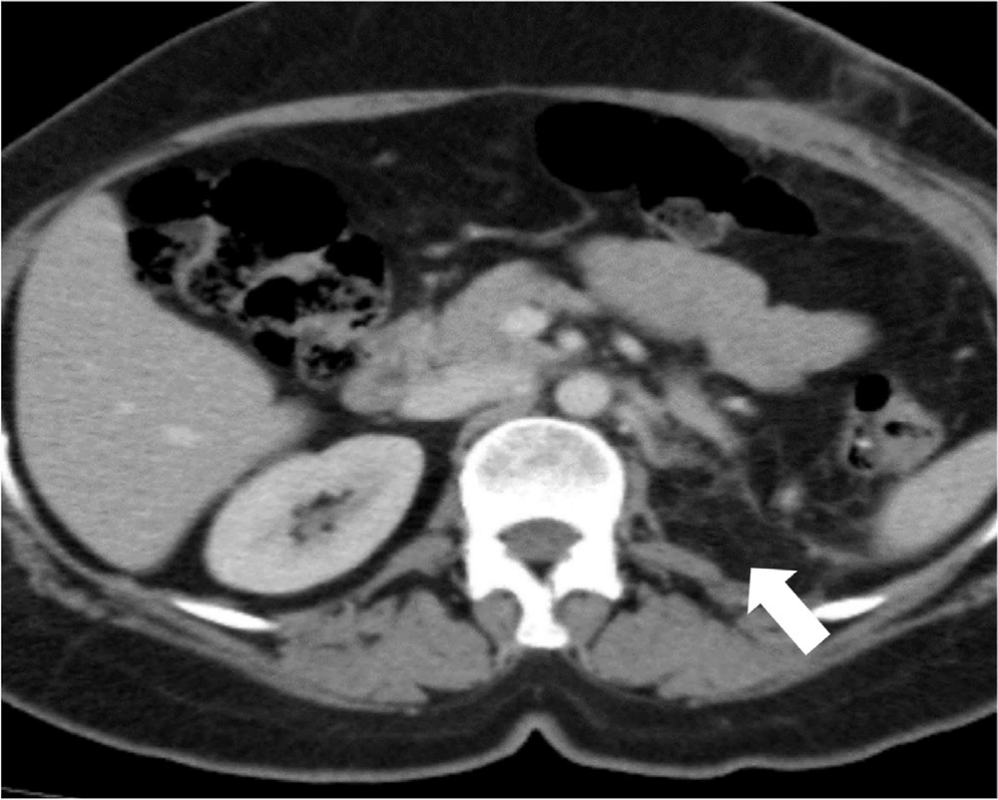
Postoperative CT scan axial image showing left nephrectomy with postsurgical changes (arrow) in left renal bed.
